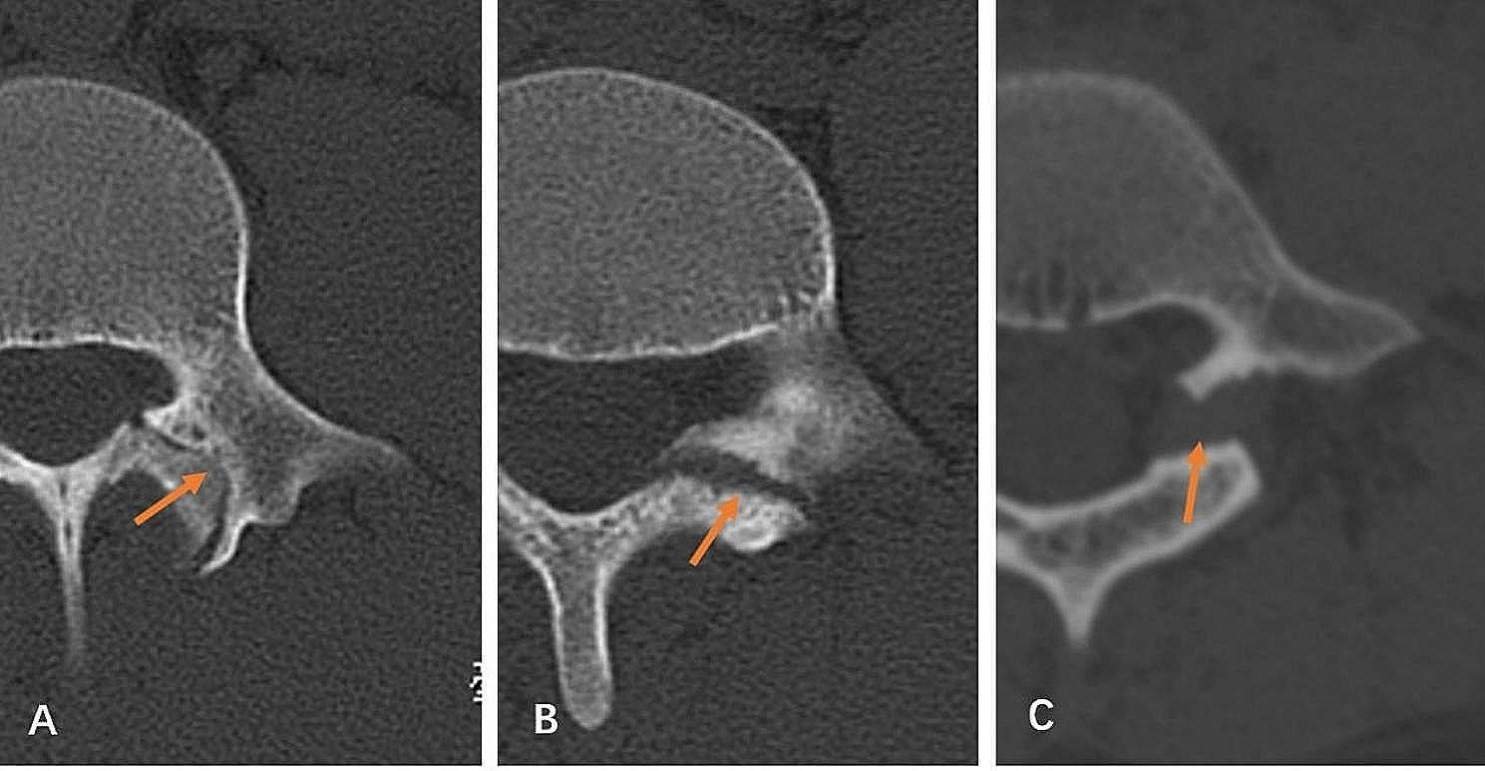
Representative CT images of different stages of pars defects (orange arrows). Stage I: Early stage, the gap is narrow, similar to hairline (A). Stage II: Progressive stage, the bone gap is clear and small fragments are present (B). Stage III: Terminal stage, characterized by a wide gap, atrophy, and sclerosis edge of the bony defect (C)
